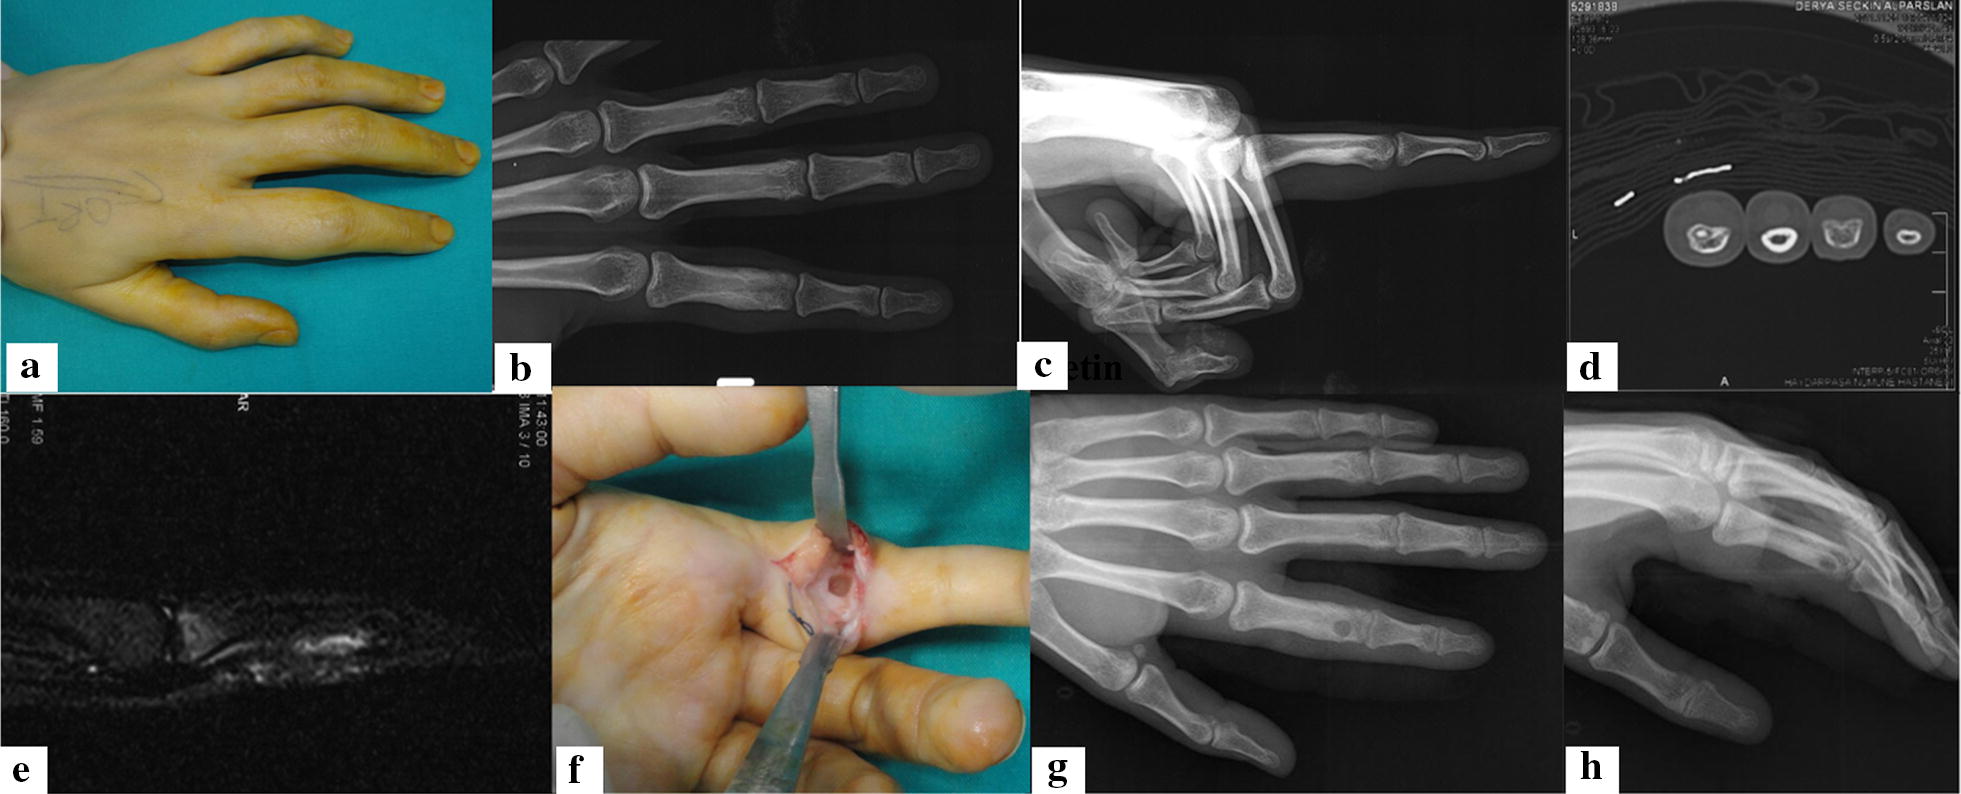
a Preoperative anterior–posterior plain graph showing cortical sclerosis. b The sole finding was volar cortical thickening in lateral view. c CT imaging axial section shows nidus formation clearly. d MR imaging sagittal section shows nonspecific edema. e Moderate swelling of the second finger was first finding. f Perioperative image shows cortical defect after curettage. g The postoperative anterior–posterior plain graph shows the cortical defect. h Postoperative lateral view shows the curettage area, possibly a little distal from the nidus center, resulting with a residual lesion
